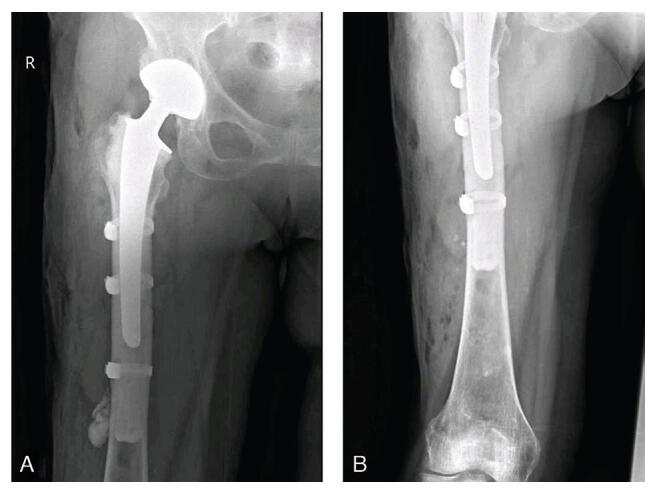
Figure 4. X-ray radiography of the hip immediately after the first revision. A, X-ray shows a piece of cement is found at the lateral of the distal femur. B, X-ray shows the piece of cement is removed.
